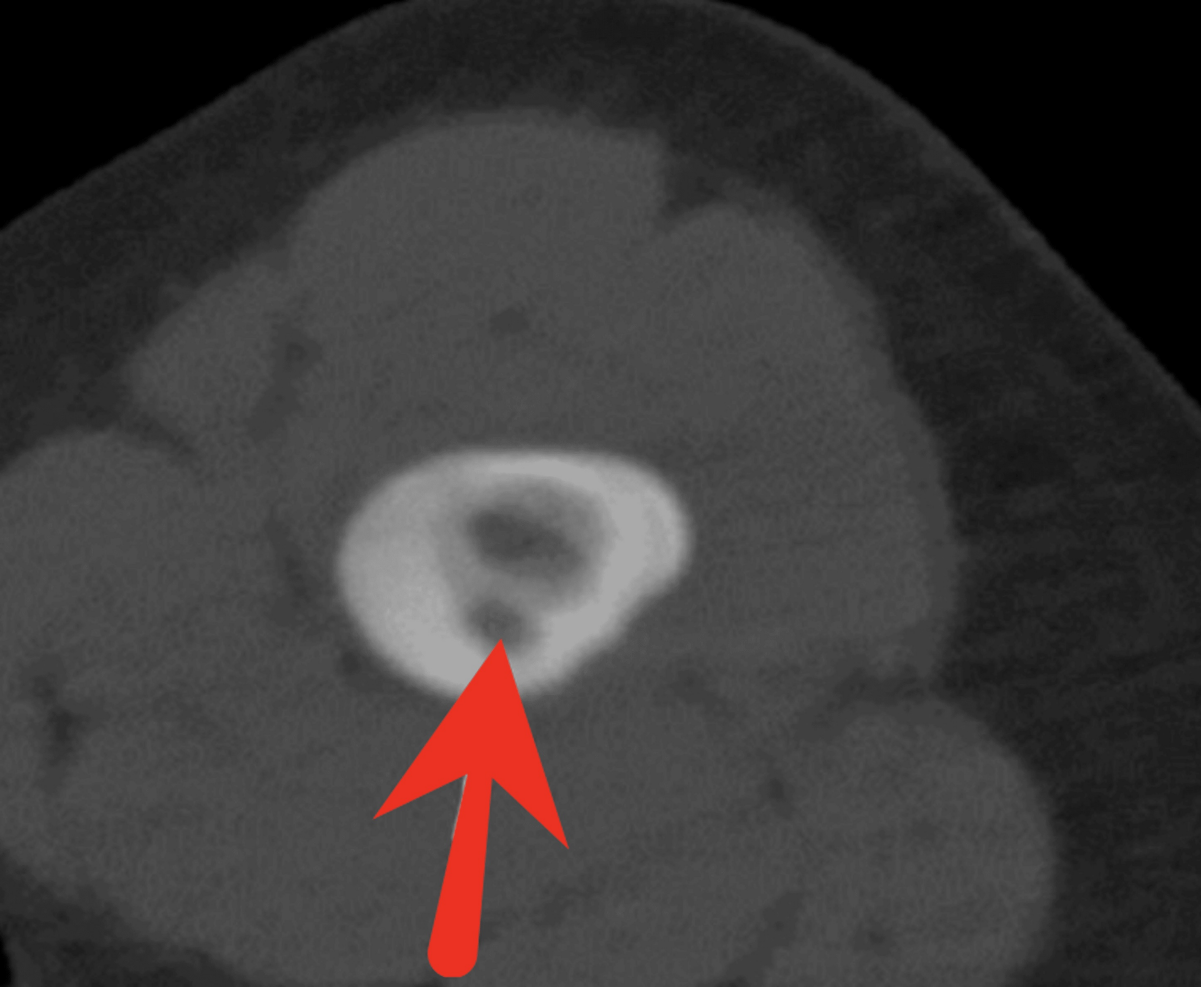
CT-Guided Radiofrequency Ablation of Osteoid Osteoma Axial intra-procedural CT image demonstrates precise placement of the radiofrequency ablation probe (red arrow) into the nidus of the osteoid osteoma located within the femoral neck. The procedure was performed under CT guidance to ensure accurate targeting of the lesion for thermal ablation. CT, computed tomography.
